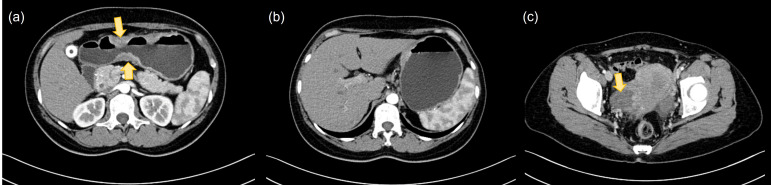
Contrast-enhanced abdominal computed tomography (CT) at initial diagnosis. (a) Imaging demonstrated advanced gastric cancer staged as T3N2, consistent with Borrmann type III (infiltrative ulcerative type). (b) Multiple morphologically abnormal small lymph nodes were observed along the lesser curvature of the stomach. (c) Bilateral ovarian enlargement was noted.
